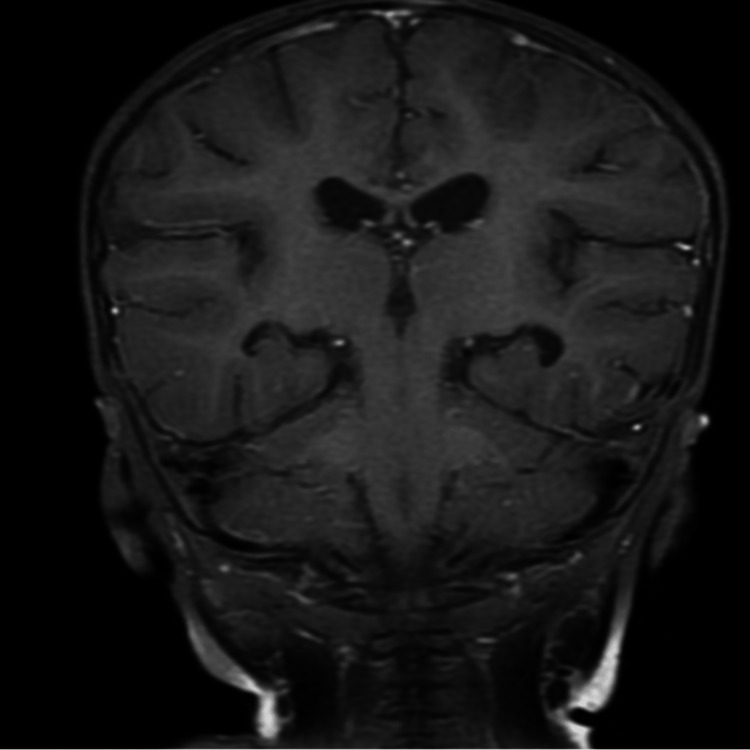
Follow-up MRI coronal view showing nearly complete resolution of the brain abscesses six weeks after treatment.
